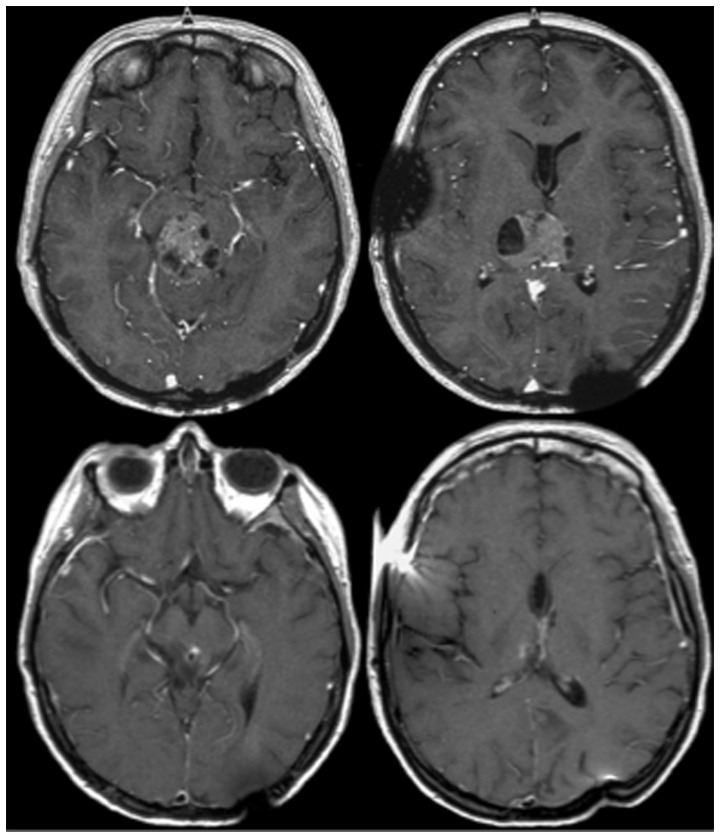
Follow-up MRI of a 14-year old female with an ependymoma III in the midbrain prior to IBT (upper line) and partial tumor remission 13 years after treatment (bottom line).
